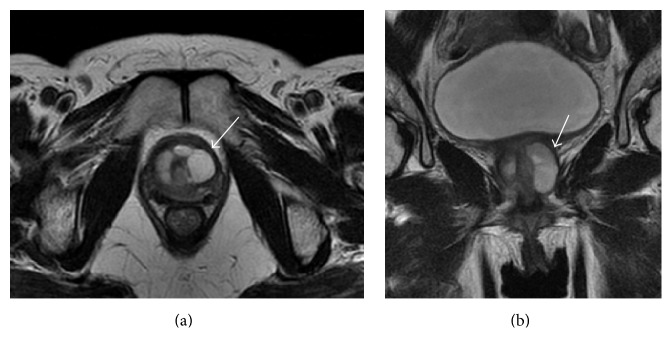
T2-weighted magnetic resonance image of the pelvis. (a) Axial and (b) coronal views of the periurethral diverticulum (arrow).
